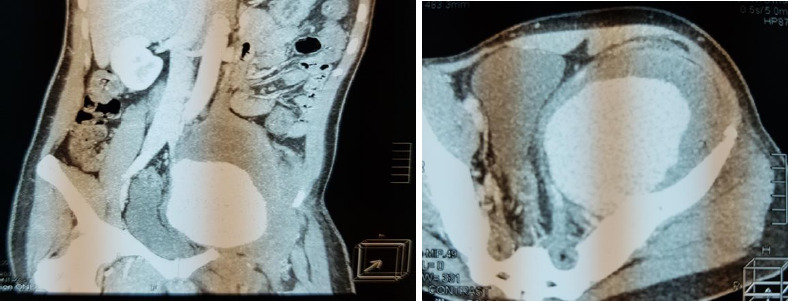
Pre-operative CT angiogram of patient 2
